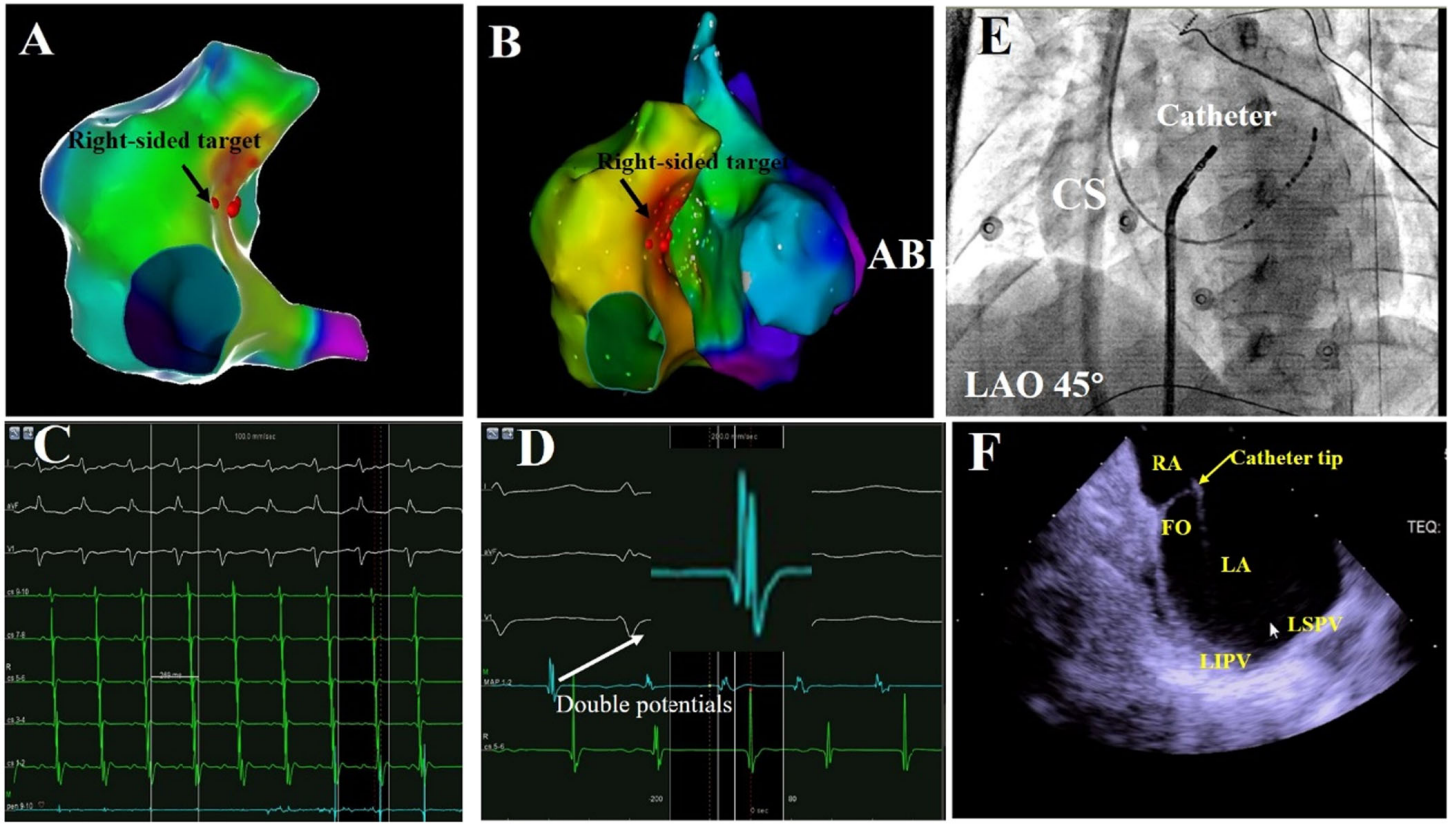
The ablation targets for a patient with FAT originating from the right‐sided FO in the Unilateral Ablation Group. (A, B) They depict the three‐dimensional electroanatomic map and the ablated target of FAT in this group. The earliest atrial activation was recorded at the right atrium of FO. (C) It provides a fluoroscopic view of the successful right‐sided ablation site of FO at the left anterior oblique 45°. (D, E) It displays the cycle lengths of FAT in intracardiac electrogram and the ablation catheter on FO with double potentials. (F) It shows intracardiac ultrasound directly demonstrating the catheter tip contact with FO.
